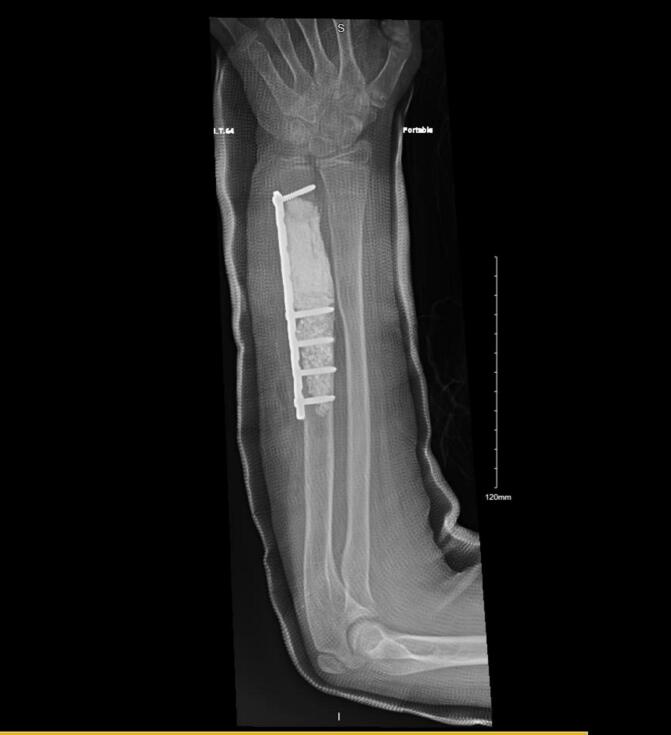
Radiograph obtained immediately after the surgery; Anteroposterior view of the left forearm demonstrating the cement, plate, and screw fixation after lesion resection.
